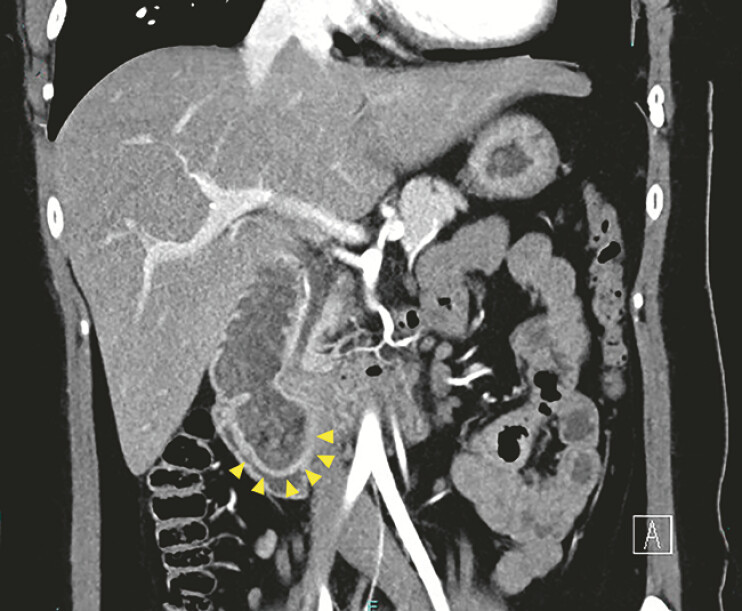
Computed tomography revealed a diverticulum in the second portion of the duodenum.
